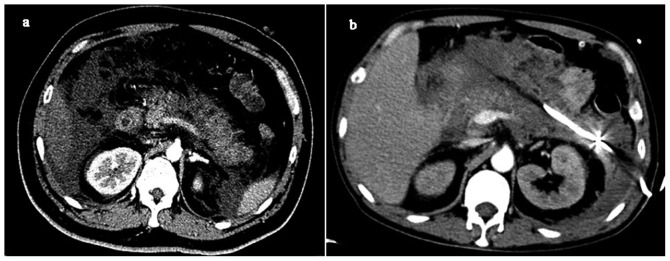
Abdominal CT scan. (a) Case 1 revealed a marked swelling and obvious pancreatic effusion. (b) Case 2 revealed a pancreatic swelling, obvious effusion and marked necrosis. CT, computed tomography.
